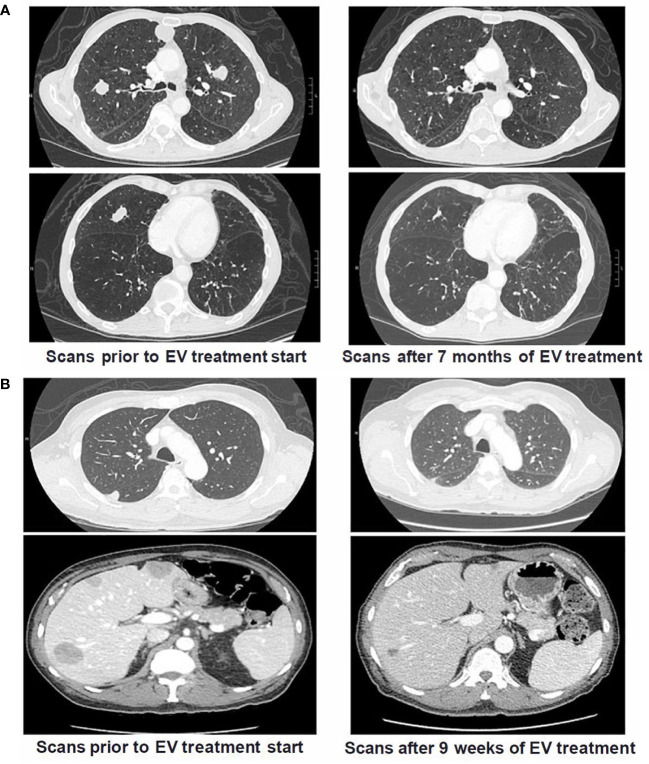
Cross Sectional Imaging of Patients with *TP53* and *MDM2* Somatic Alterations Pre and Post Treatment with Enfortumab Vedotin. (A) Patient with metastatic UC and *TP53* alterations (p.E285* and p.E271Q) who had prior progression on both platinum-based chemotherapy and pembrolizumab. Patient had significant decrease in size of biopsy-confirmed pulmonary metastases after starting treatment with EV and eventually achieved a complete response. Patient continues to have radiographic CR on scans 30 months after EV treatment start. (B) Patient with metastatic UC and *MDM2* alteration (amplification) who had prior progression on a clinical trial of an immune checkpoint inhibitor combination regimen with metastases in lungs and liver. Following start of EV treatment, patient had marked reduction of metastatic burden after only 9 weeks and continued on treatment for >18 months.
